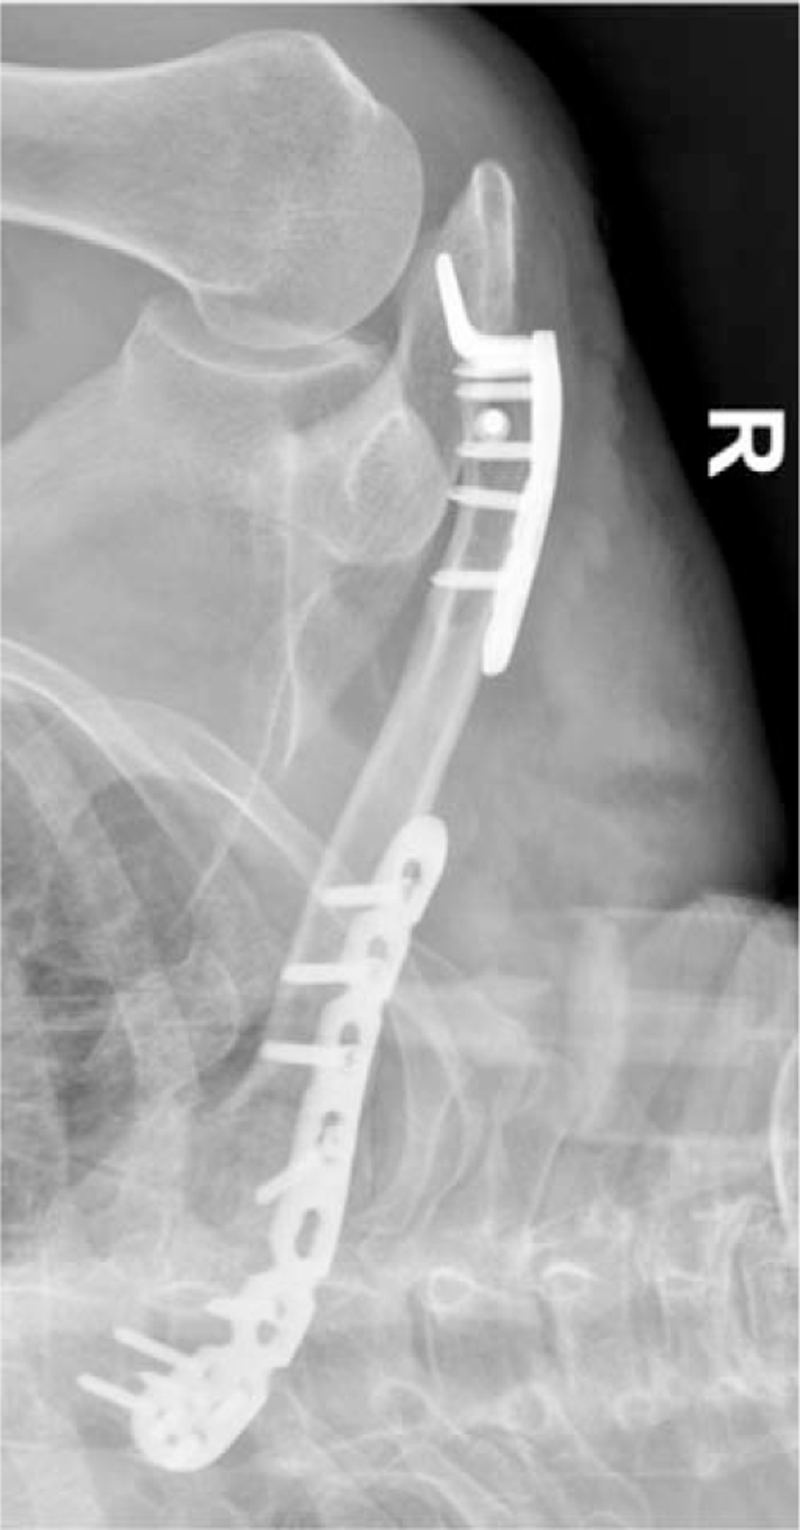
Postoperative X-ray shows the medial fracture trans-sternoclavicular locking plate fixation and lateral fracture hook plate fixation.
